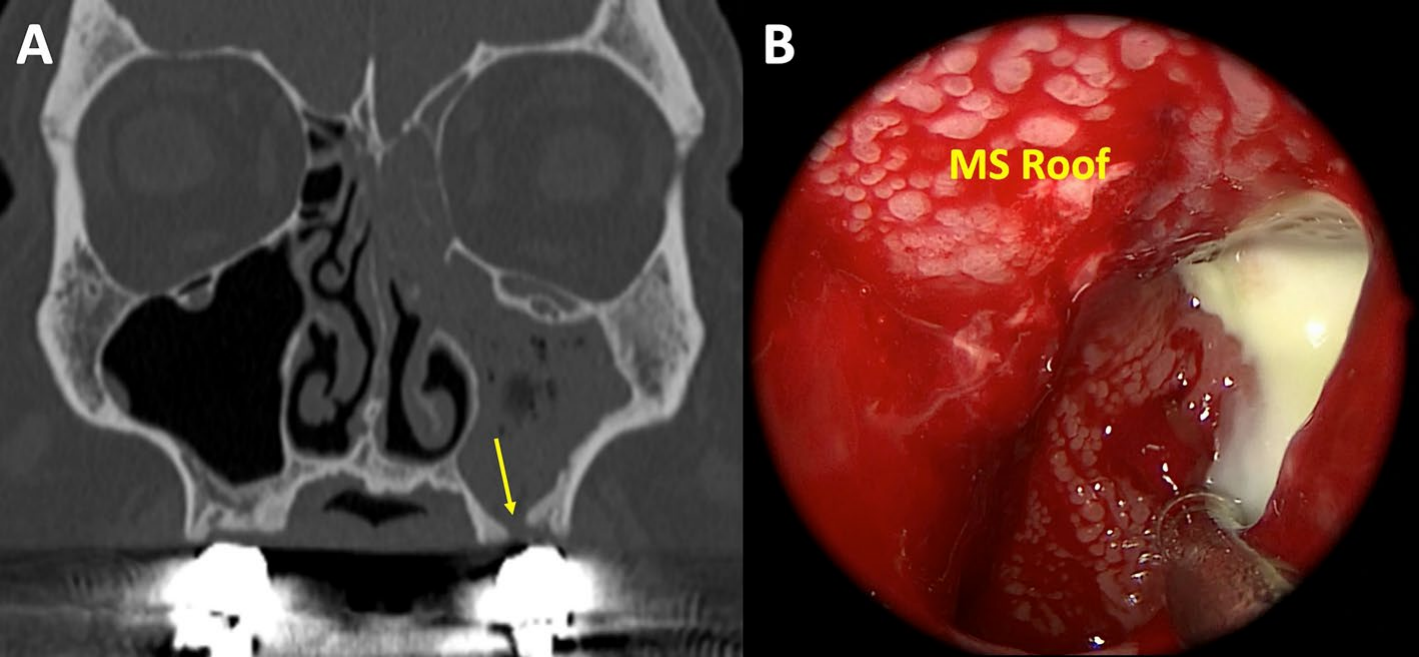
Characteristic example of odontogenic sinusitis. (A) Computed tomography showing left‐sided maxillary and ethmoid sinus opacification with bony dehiscence of the maxillary sinus (MS) floor after prior molar extraction and bridge placement (yellow arrow). (B) 70° nasal endoscopic view of the left MS lumen after wide maxillary antrostomy, demonstrating characteristic diffuse papillary edema of all the MS walls.
